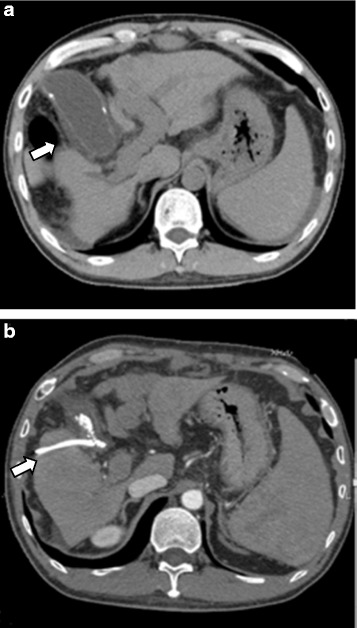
a–b. Pretransplant abdominal computed tomography CT imaging studies in our case 2 showed gallbladder distention and wall thickness before cholecystostomy (*arrow* in a), and cholecystostomy (*arrow* in b) resolved these findings
